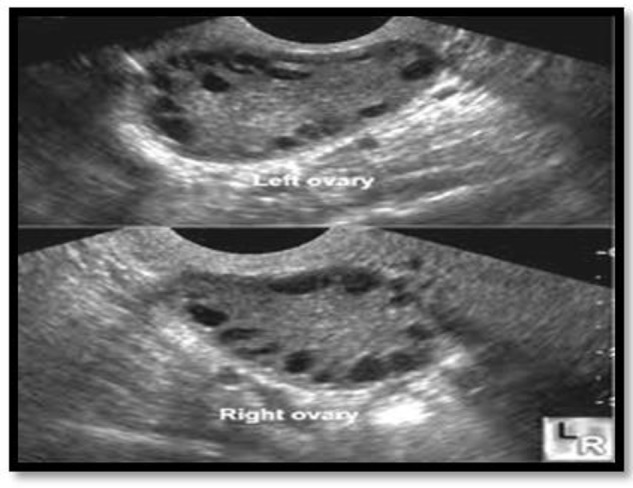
This sonography displaying polycystic ovaries
